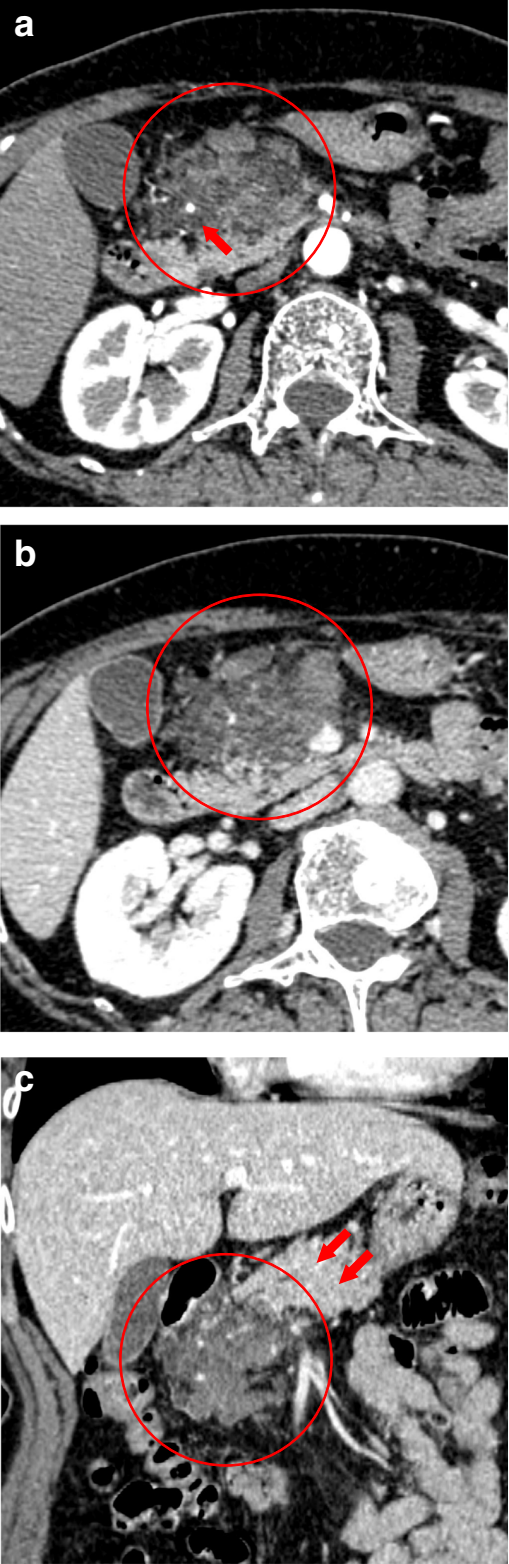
The contrast-enhanced dynamic CT scan showed a 60-mm-diameter tumor in the pancreatic uncinate process (red circle in each image). a Arterial phase (red arrow: gastroduodenal artery). b, c Portal vein phase (red arrow: normal pancreas)
